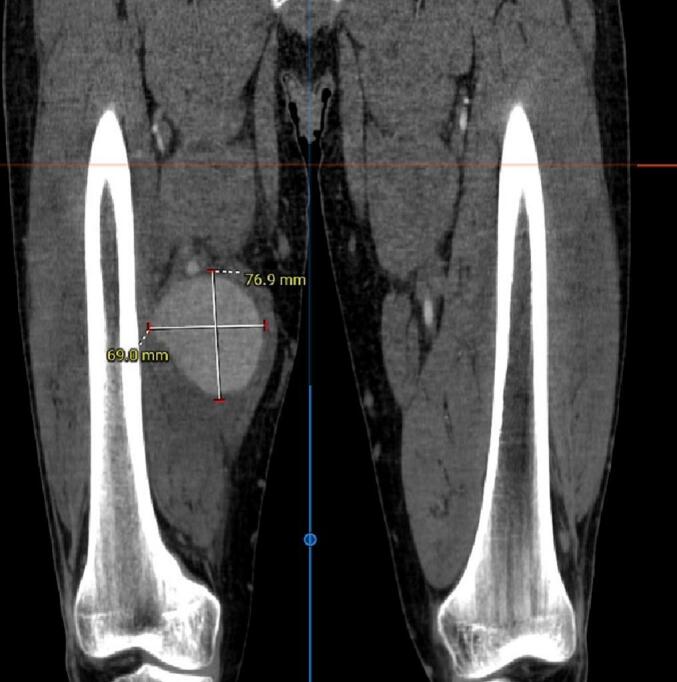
A CT angiography of the lower extremities: Coronal view demonstrating a giant pseudoaneurysm of the right superficial femoral artery (SFA) with a diameter of 76.9 × 69.0 mm.
